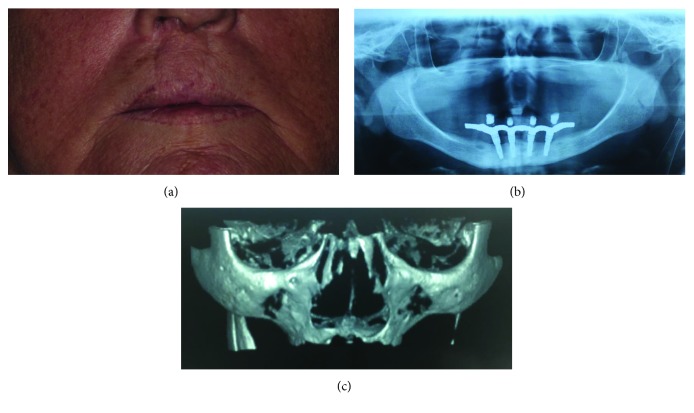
(a) Preoperative clinical condition of the patient, (b) preoperative panoramic radiography, and (c) preoperative cone beam computed tomography. Note the large degree of maxilla atrophy presented by the patient before the surgical procedure.
